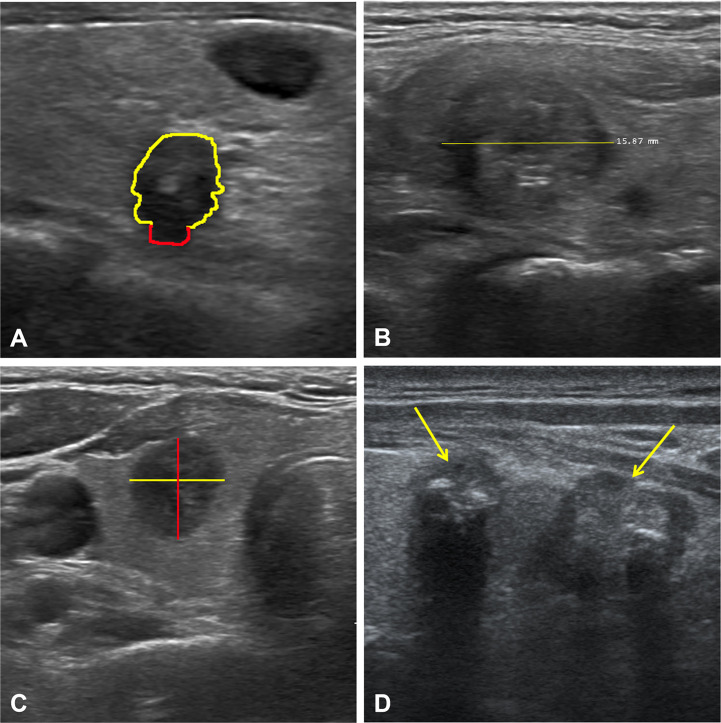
Ultrasound images of four representative cases. Case 1 (A): The nodule with extrathyroidal extension (red line). Case 2 (B): A solid nodule with a diameter of 1.6 cm. Case 3 (C): A hypoechoic nodule with taller-than-wide. Case 4 (D): Multifocality in the left lobe of the thyroid (yellow arrows). Together, all these features were the most essential factors in the diagnostic performance of the RF algorithm based on ultrasound features. RF, random forest.
